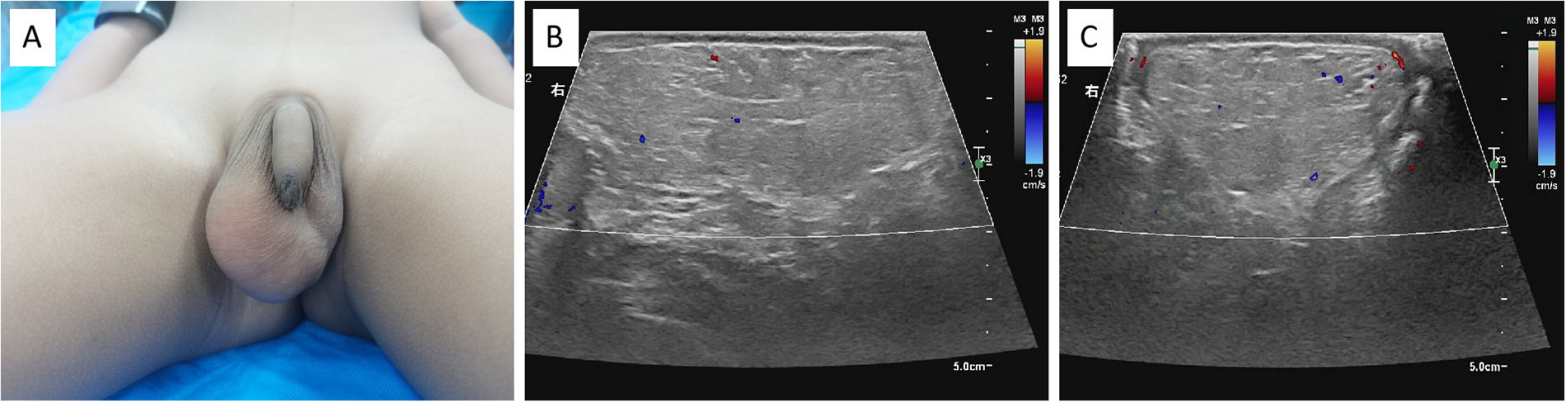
(A) A lipoma can be seen in the right scrotum of the child. (B) The ultrasound revealed a hypoechoic lipoma in the right scrotum with a clear boundary (transverse section). (C) The ultrasound showed the longitudinal section of the scrotal lipoma.
